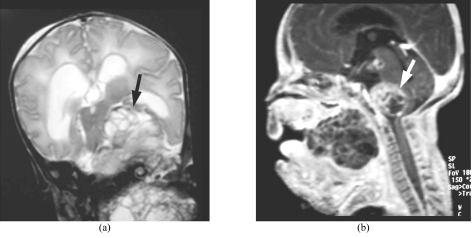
MR images two weeks after removal of the teratoma: (a) T2 weighted (TR 4500, TE 94) in coronal plane; and (b) T1-weighted (TR 9.7, TE 4.1) contrast-enhanced sequence in sagittal plane, showing recurrence. Tumour is seen occupying the oral cavity with extension into the left temporal fossa (black arrow) and brain stem compression (white arrow). There is evidence of moderate hydrocephalus.
